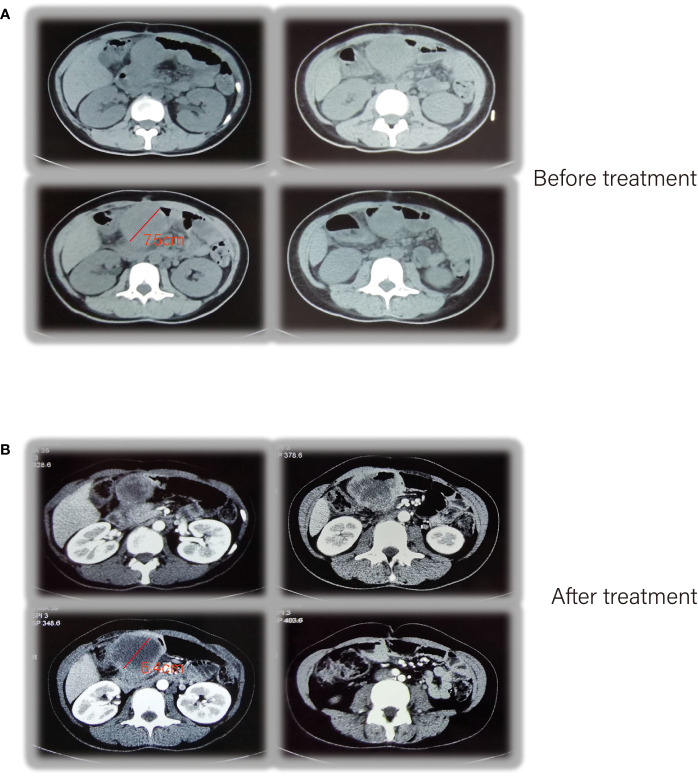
CT enhancement of the abdomen showed tumor recurrence (A), after receiving pembrolizumab therapy (B).
